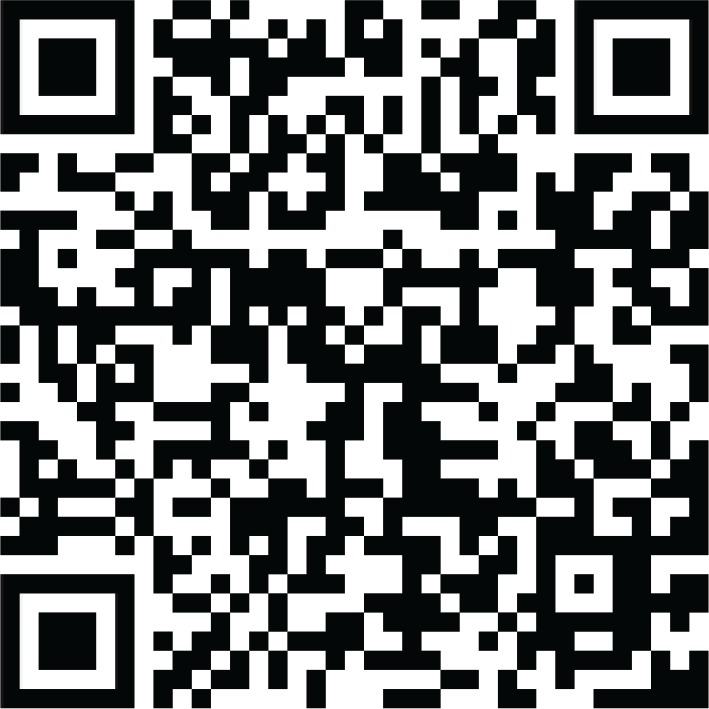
Transthoracic echocardiography, Doppler evaluation: bidirectional flow between the left ventricle and the left ventricular pseudoaneurysm cavity.
